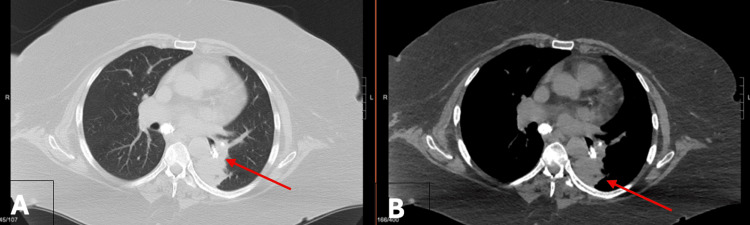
Computed tomography findings of broncholithiasis in Case 2. Axial contrast-enhanced chest CT images displayed in lung window (A) and mediastinal window (B) demonstrate calcified mediastinal lymphadenopathy with broncholiths obstructing the left lower lobe bronchus (arrows). Associated post-obstructive changes are evident, correlating with the patient’s clinical presentation of hemoptysis and recurrent infection.
